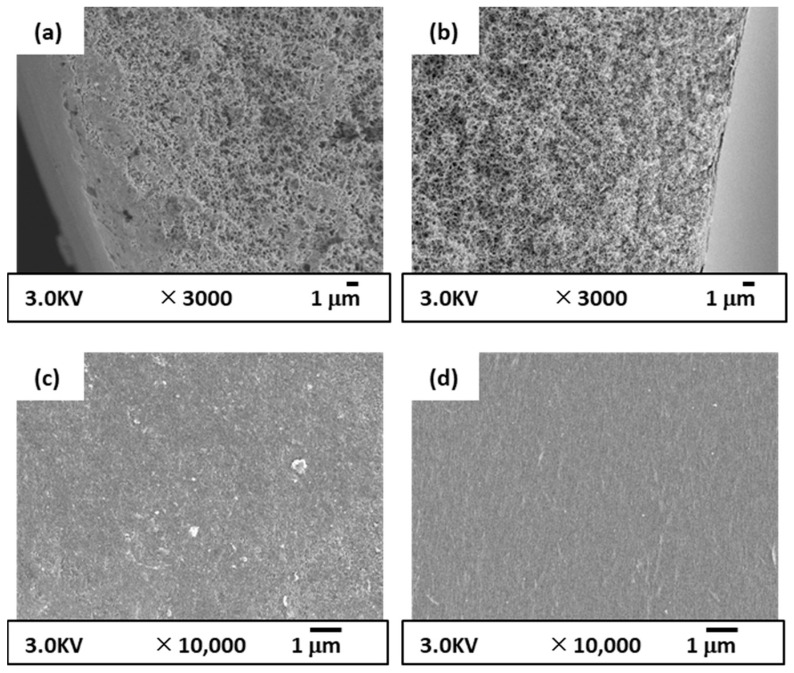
SEM images of prepared CTA hollow fiber membranes prepared by NIPS method; (a): cross-section near the outer surface (b): cross-section near the inner (c) outer surface (d) inner surface.
